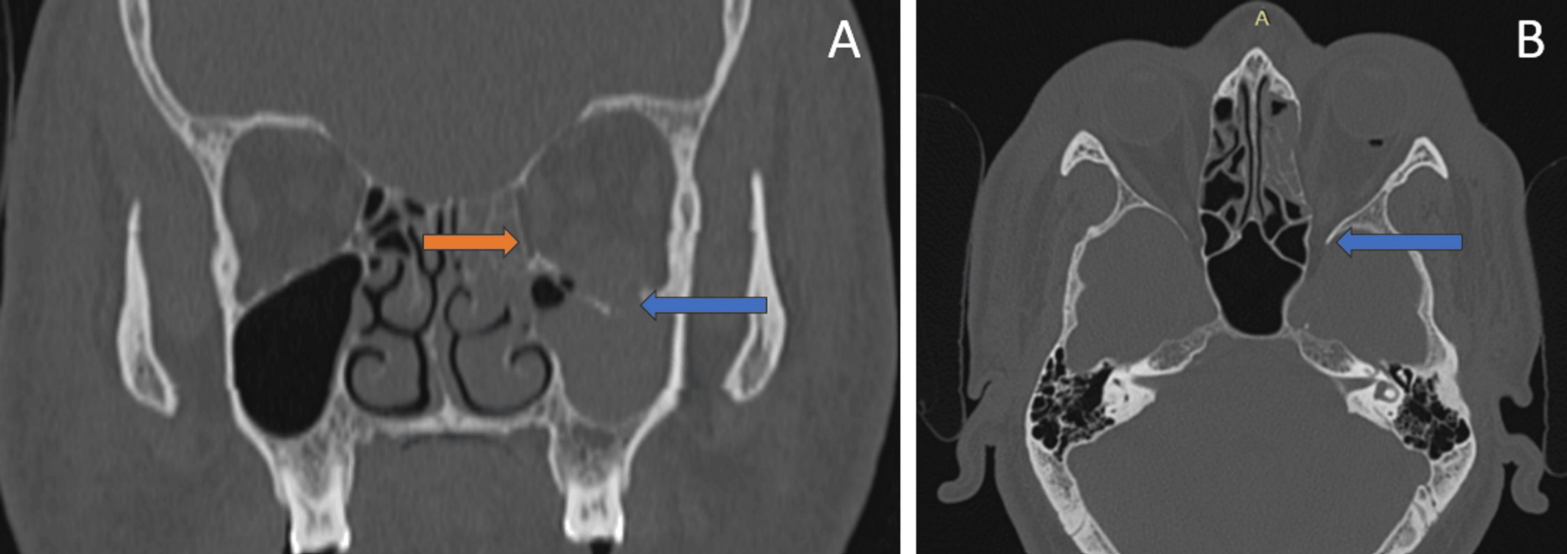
(A) Coronal non-contrast computed tomography image of the orbit shows fracture of the floor (blue arrow) and medial wall (orange arrow) of the left orbit. (B) No fracture of the left optic nerve canal (blue arrow).
